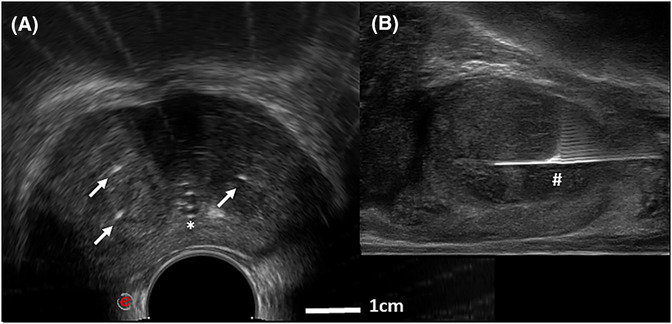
(A) Example of a transversal plane ultrasound image of the prostate. An indwelling catheter is placed for urethra identification (above the asterisk), and three needles are placed (arrows). (B) Sagittal plane view of the same prostate. The fibre is introduced into the needle, extending 1 cm from the needle tip (hash).
